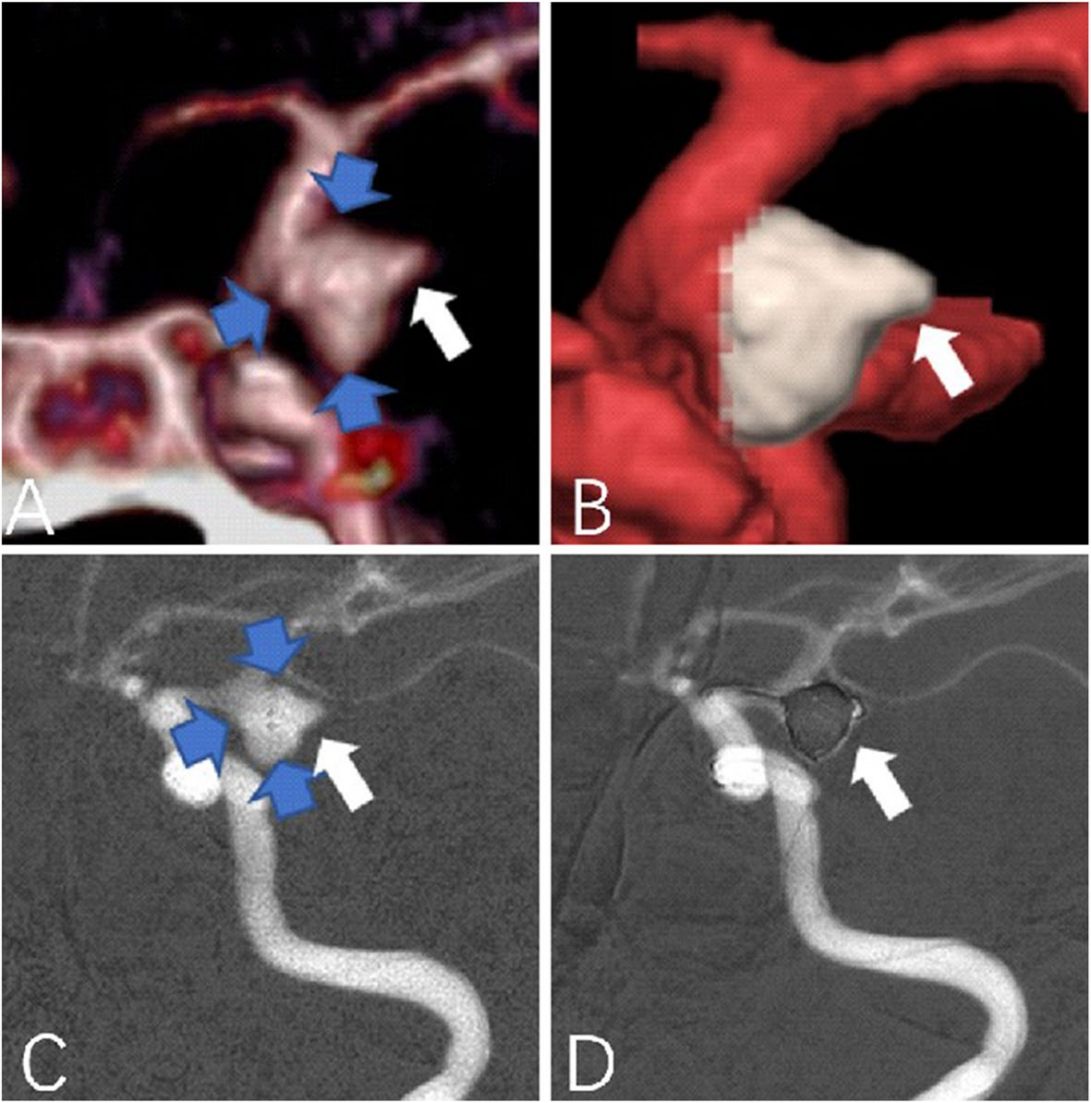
A-B CTA showed a right posterior communicating artery aneurysm (PcomA) aneurysm (5.5 mm x3.8 mm), and an obvious pulsation was observed by 4D-CTA (as shown by the white arrow). Both observers recorded the aneurysm as small, having more than two lobes (as shown by the blue arrow), a narrow neck, and clear visualization of parent and branch vessels. C-D DSA findings confirmed CTA findings, the patient underwent interventional embolization therapy after a 36-day follow-up finally in frame D
